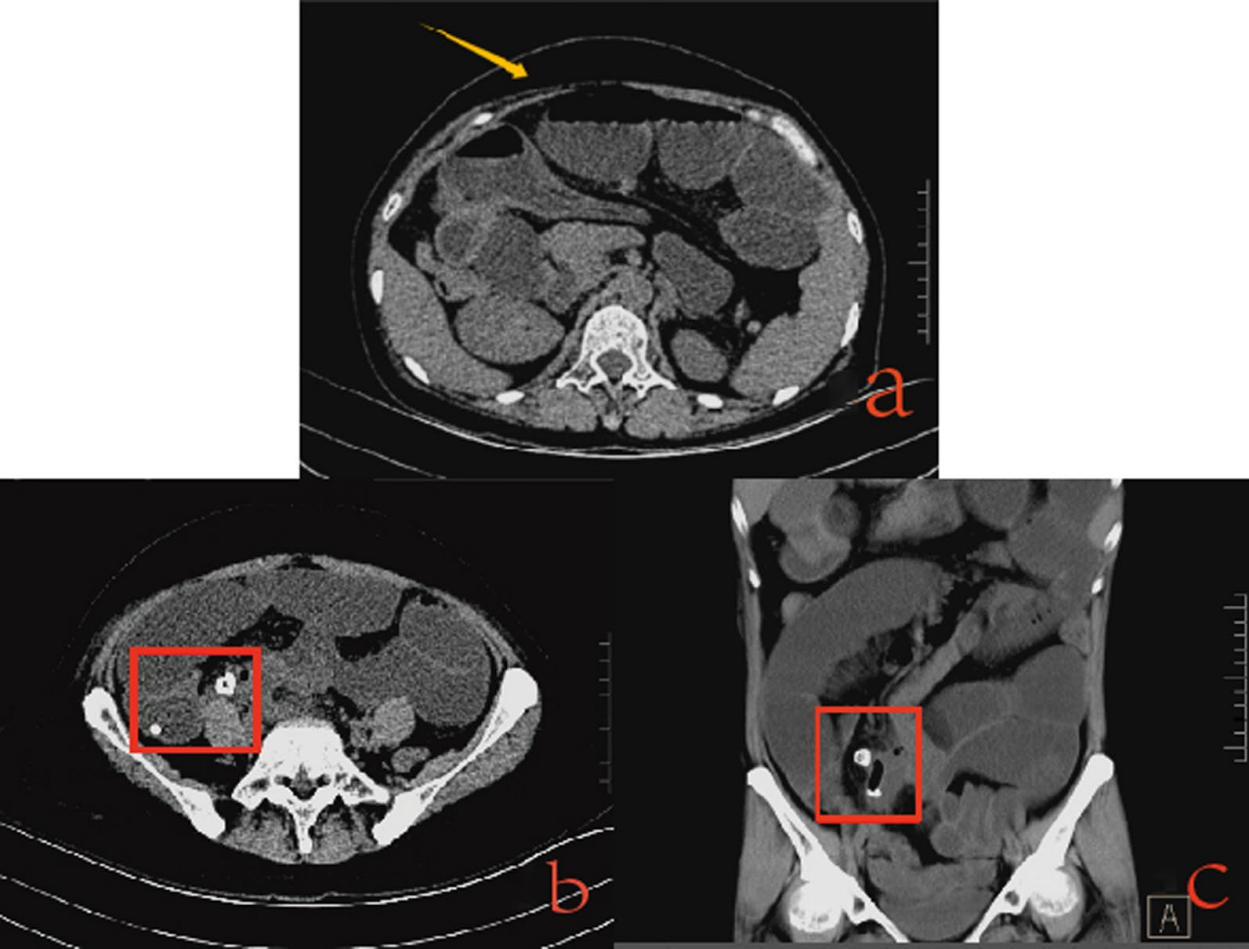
a Computed tomography (CT) of the abdomen showed partial small bowel dilatation with a gas–liquid level inside. b Cross section Computed tomography (CT): Increased density of the local intestinal canal in the right lower quadrant. c Coronal Computed tomography (CT): increased density of the local intestinal canal in the right lower quadrant
